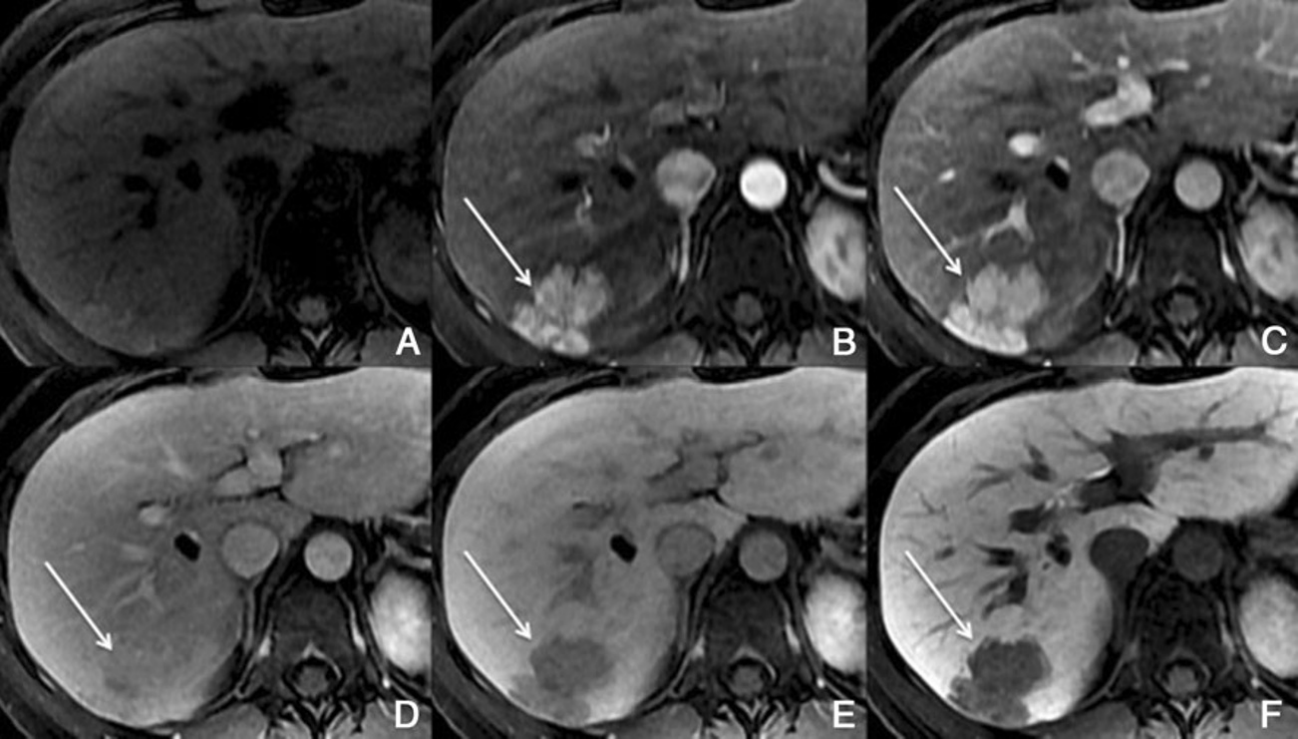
Liver adenoma in a 35-year-old woman on oral contraceptives, with no liver disease. a Pre-contrast MRI, b, c arterial phase, d 3 min, e 5 min, f HCP. Lobulated lesion in the right lobe isointense in pre-contrast imaging, with arterial enhancement, internal arteries and no uptake of Gd-EOB-DTPA in the HCP (*arrow*)
